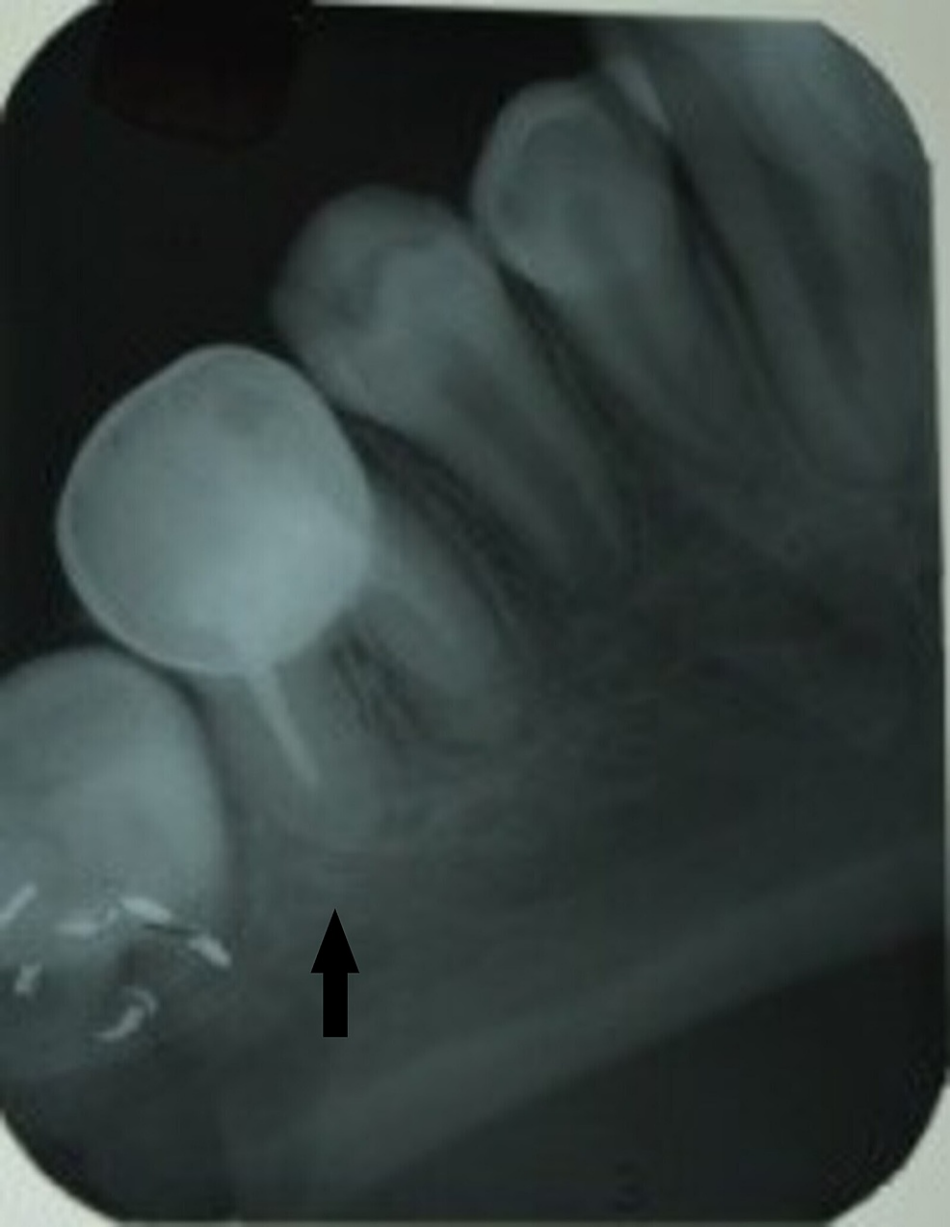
One-year follow-up - IOPA radiograph IOPA: intraoral periapical
